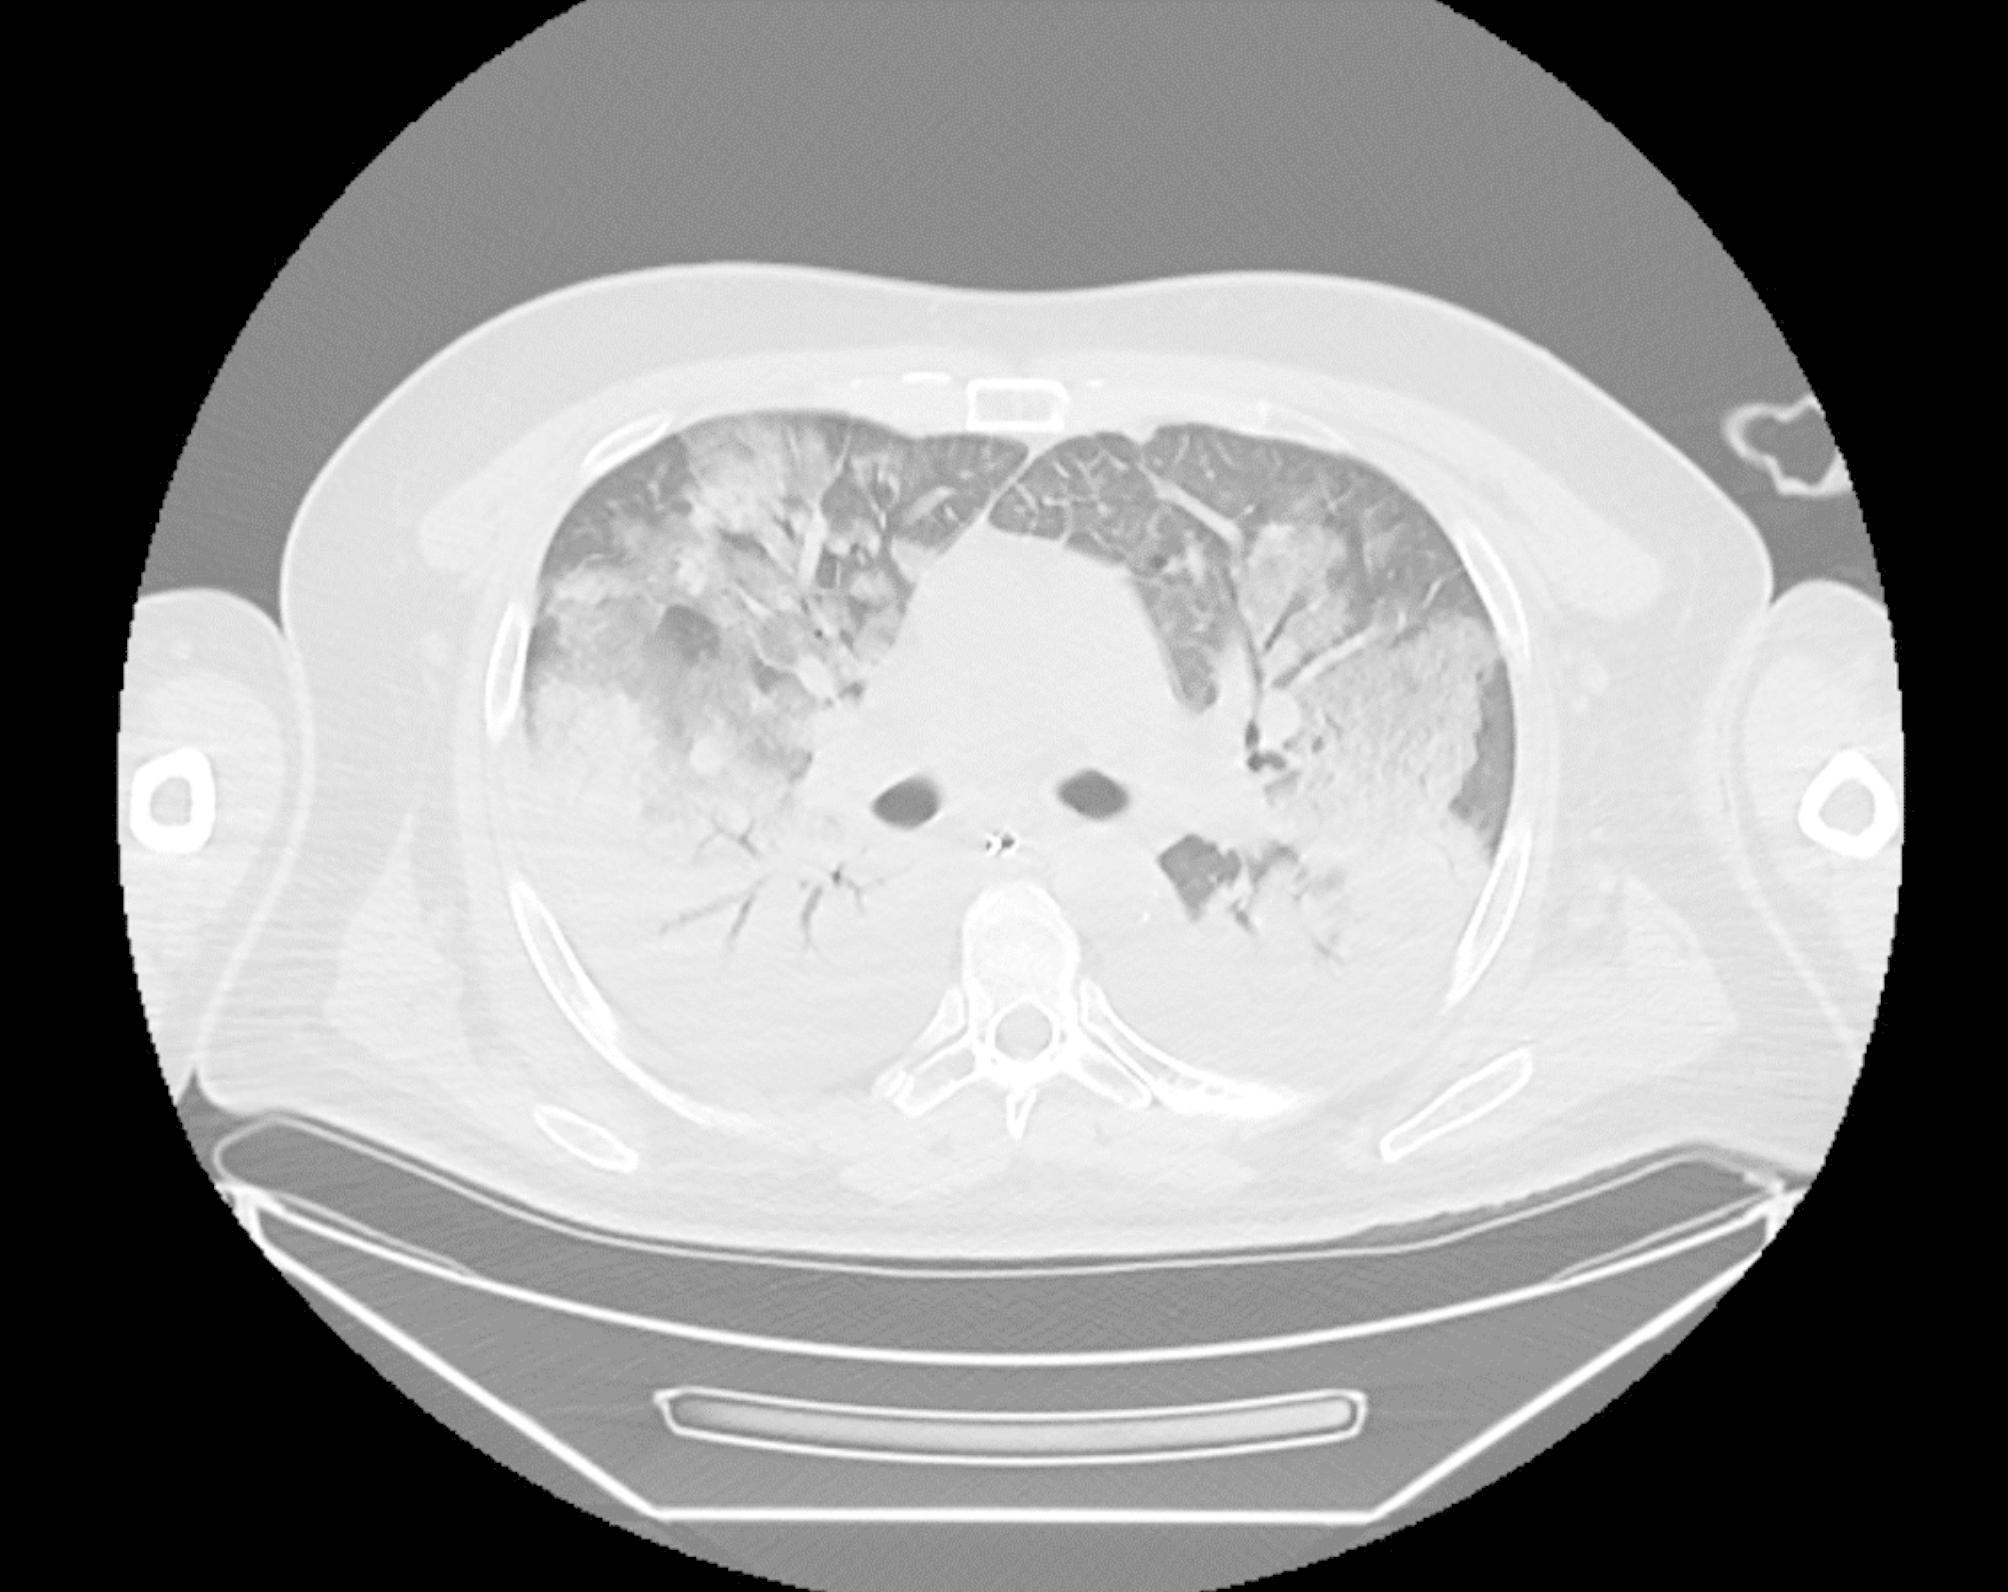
Chest computed tomography on admission
